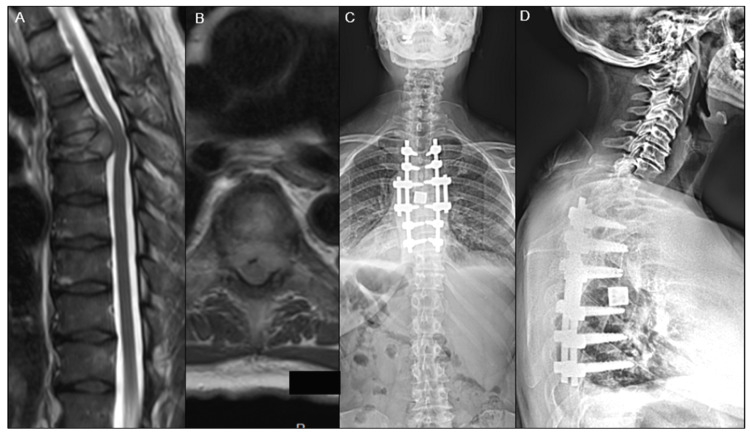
A 53-year-old female presented to the clinic with a several-week history of back pain and difficulty ambulating due to poor balance. On preoperative T2-weighted MRI (A,B), the patient was found to have T6 Bilsky grade 3 epidural spinal cord compression due to metastatic breast cancer. The patient underwent separation surgery with T3-T9 posterolateral fusion, T5-T7 laminectomies, T5-T6 and T6-T7 osteotomies, inferior facetectomies at T3-T9, costotransversectomy on the left side at T6 with removal of 4 cm of the left T6 rib, and bilateral transpedicular decompression at T6 with T4-T7 anterior fusion with a static cage filled with allograft and demineralized bone matrix, as seen on postero-anterior (C) and lateral X-ray (D).
